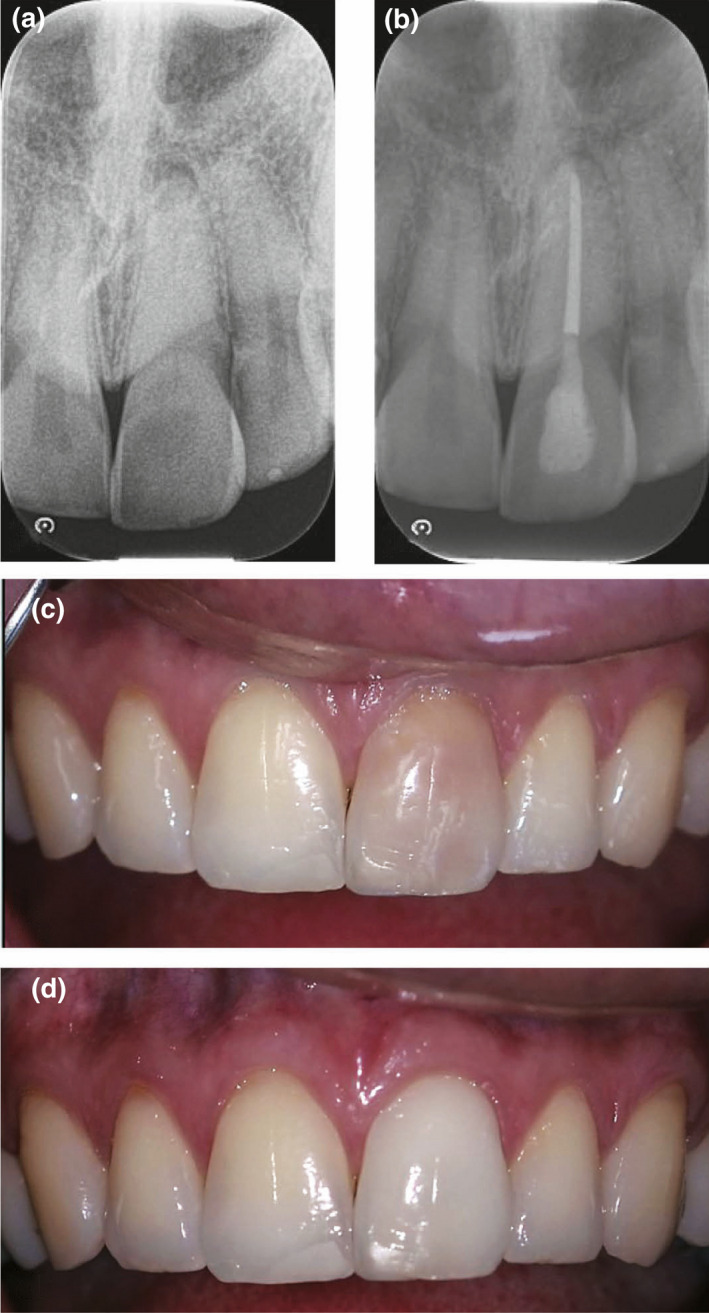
(a) A preoperative radiograph showing a left maxillary central incisor calcified canal with an obliterated canal and a periapical radiolucency. (b) A periapical radiograph of the tooth root filled. (c) A clinical radiograph revealing a brownish discolouration. (d) A clinical photograph of the tooth treated in three appointments using the bleaching protocol as described in Figure 5
